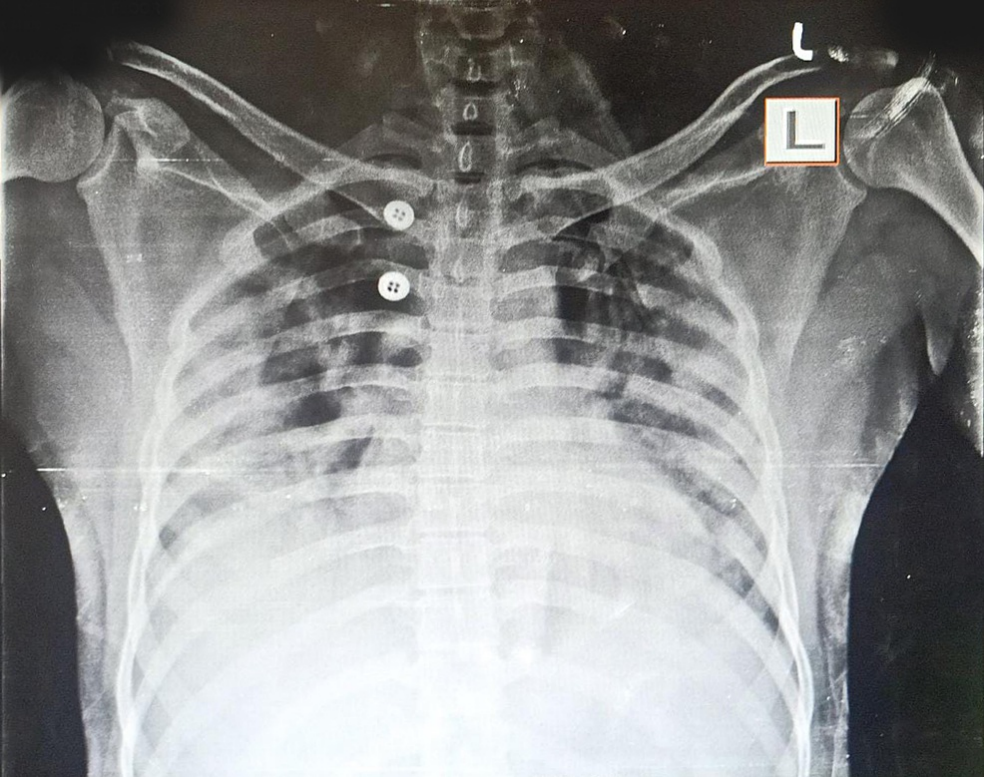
X-ray of case 2 after bevacizumab
